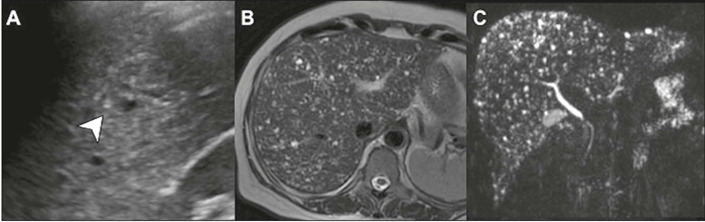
Biliary hamartomas. Ultrasound (A) showing small cysts
